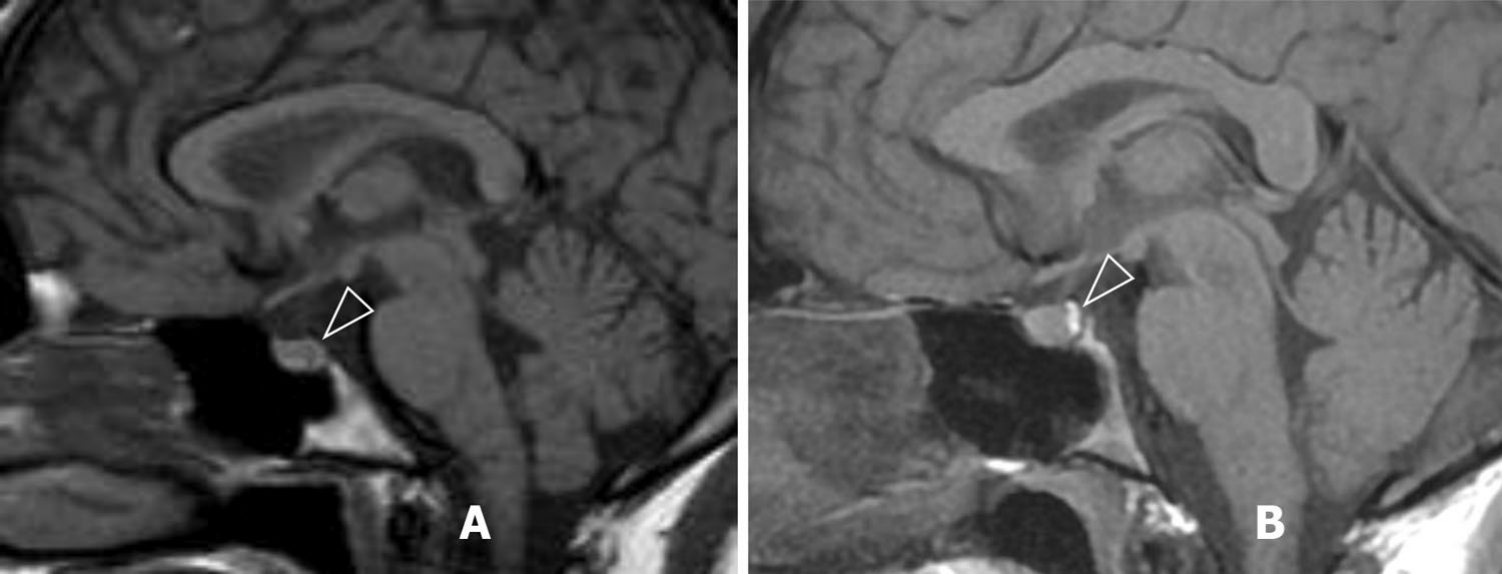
T1-weighted MR imaging revealed a reduced bright signal from the posterior pituitary gland in case 9 (A, arrowhead) in comparison to the normal bright signal in case 4 (B, arrowhead)
